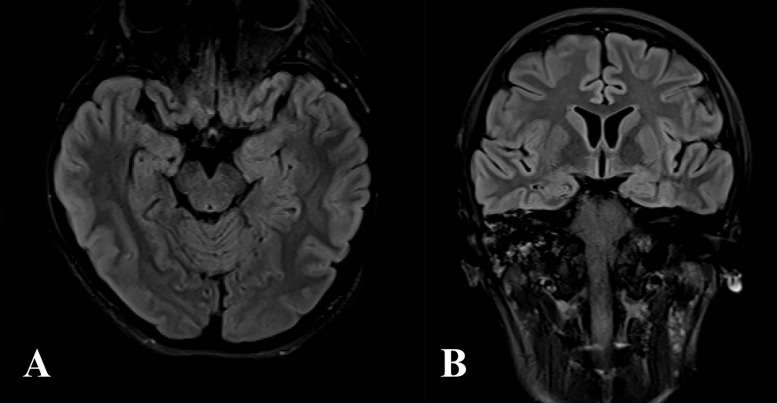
(A) T2-weighted magnetic resonance imaging (MRI) of the head at time of discharge, demonstrating improvement of the previously documented increased signal intensities along the inferior aspect of the left temporal lobe and the insular cortex on axial and (B) coronal views.
